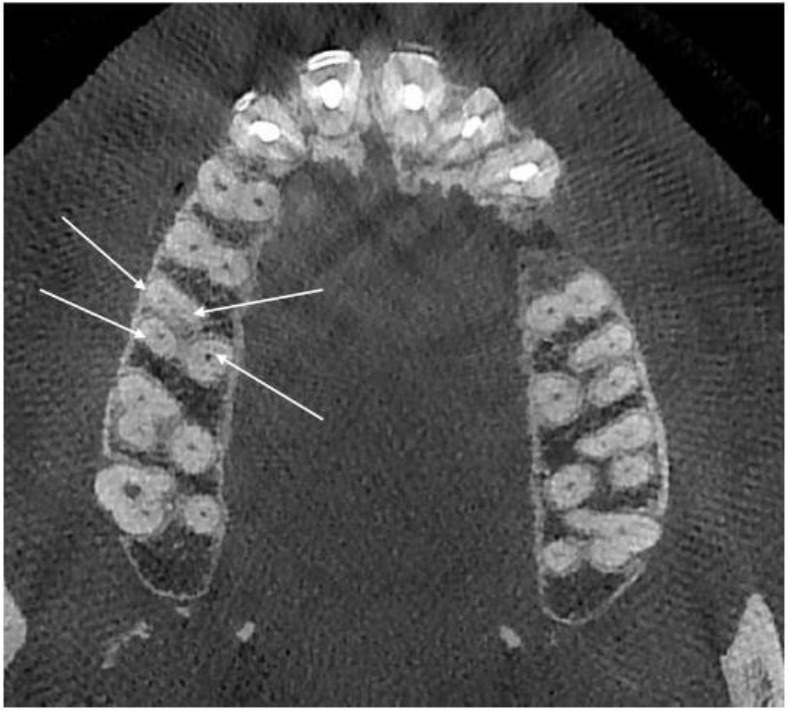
Assessment of the number of roots and their morphology and the number of canals and their configuration on CBCT scan
